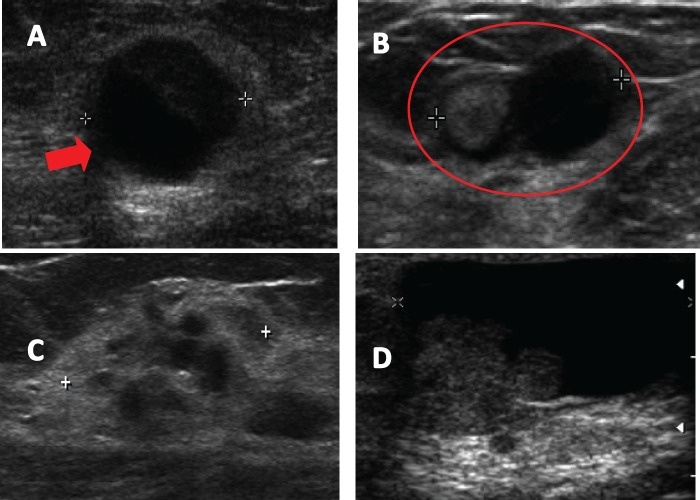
Ultrasound images described in breast papillary lesions. A. Complex solid cystic nodule. B. Lactiferous duct dilated hypoechoic content. C. Poorly defined hypoechoic nodule. D. Cystic lesion with mural pedunculated mass.
